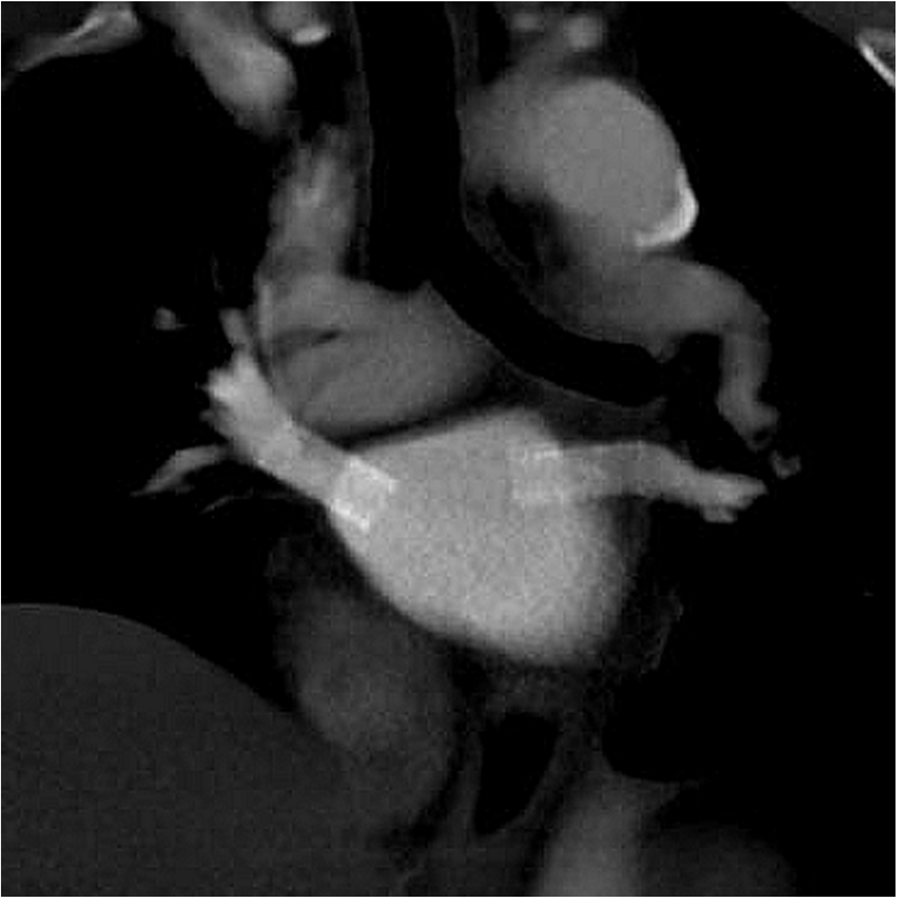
Oblique coronal maximum intensity projection CT showing no restenosis of the two PVs at 30-month follow-up
